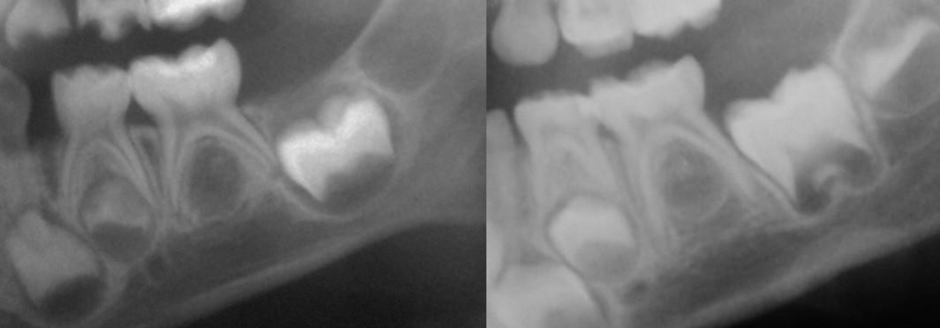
Radiographic example of modal stages of P1, P2 and M2 at P2 stage ‘Ci’ (left) and an individual with P2 stage ‘Ci’ relatively late (right). The modal stages were P1 ‘Coc’ and M1 ‘Crc’. In the case of late initiation, stages were P1’C3/4’ and M1 ‘R1/4’.
